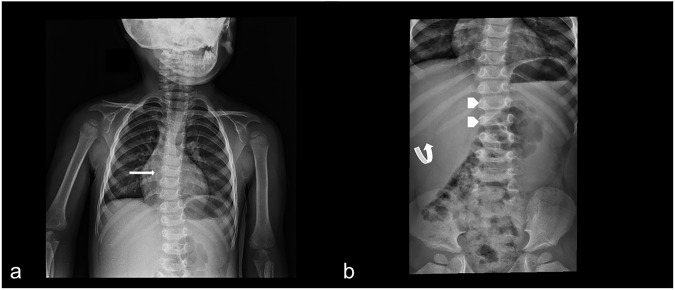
X-ray examination, spine and thorax studies. A pediatric patient with mental and developmental illness, suffering from mucopolysaccharidoses. a A scoliotic attitude of the thoracolumbar spine is well depicted (white arrow). Widened and extended-looking ribs, especially the lower one, could be observed in a and b (curved arrow in b). Vertebral bodies are rounded and flattened (white arrowhead)
